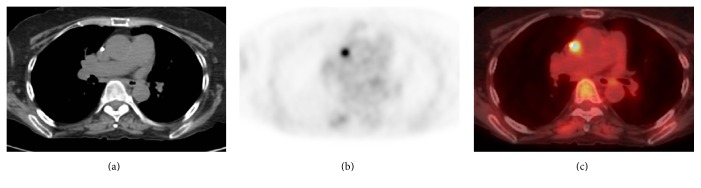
FDG PET/CT exam. Axial CT (a), PET (b), and (c) fused images from PET/CT exam demonstrate moderately hypermetabolic focus correlating with the catheter tip on CT.
